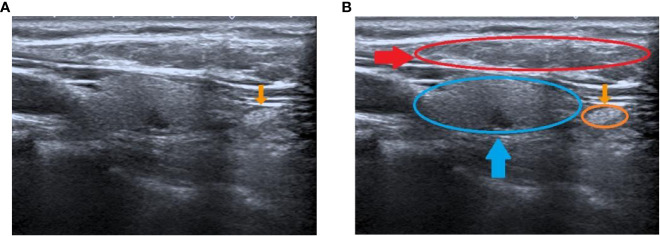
Preoperative ultrasound identification of the IPG. (A) yellow arrow shows the IPG; (B) yellow arrow and circle show the IPG, blue arrow and circle show the thyroid, red arrow and circle show the muscle.
